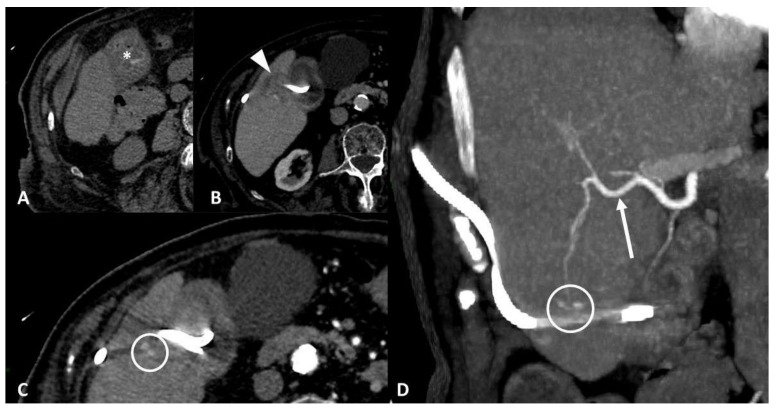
Vascular injury. Seventy-five-year-old patient affected by bleeding after percutaneous cholecystostomy. Axial non-enhanced CT scan (A) shows hyperdense blood-like material inside the gallbladder (*). Contrast-enhanced CT scans (B,C) show liver parenchymal laceration (arrowhead) in segment V, next to gallbladder, with two hyperdense spots inside. Maximum intensity projection coronal image (D) shows more clearly two pseudoaneurysms of a distal branch of the right hepatic artery (arrow) along drainage course.
